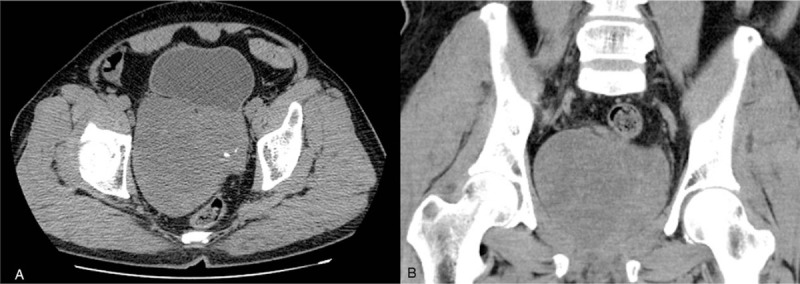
CT imaging of the GMPC. Axial (A) and coronal conventional CT scan reveals a multilobulated soft tissue mass in the pelvic with low-density area in the tumor. GMPC = giant multilocular prostatic cystadenoma, CT = computed tomography.
